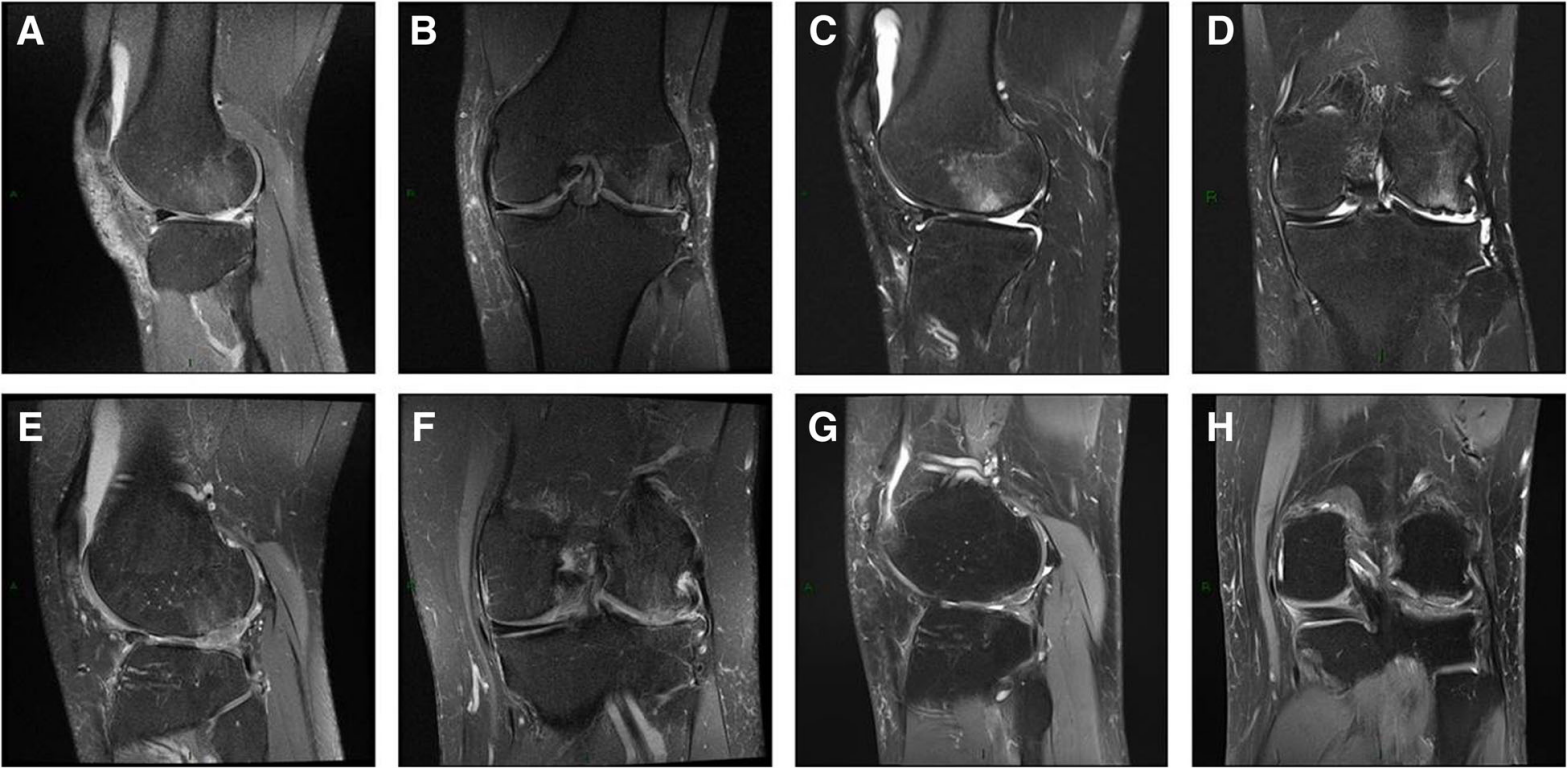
MRI post-surgery. Patient SPC: a sagittal fat-supressed proton density-weighted image 3 months after treatment. The graft tissue homogeneous filling the defect. Subchondral marrow edema. b Coronal fat-suppressed proton density-weighted image 3 months after treatment. Graft tissue homogeneous filling the defect. There is subchondral bone marrow edema, C: Sagittal fat-suppressed proton density-weighted image 53 months after treatment. The repair site is now filled with a congruent articular surface. Normal signal of the cartilage. Persistent bone marrow. d Coronal fat-suppressed proton density-weighted image 53 months after treatment. The repair site is now filled with a congruent articular surface. Persistent bone marrow edema and a focal irregularity in superficial layer are present. Patient CRC: e Sagittal fat-suppressed proton density-weighted image 6 months after the index procedure. The graft tissue inhomogeneous fills the defect with overgrowth of repair cartilage. Subtle subchondral marrow edema. f Coronal fat-suppressed proton density-weighted image 6 months after treatment. There is graft tissue in homogeneously filling the defect with overgrowth of the repaired cartilage. Subtle subchondral marrow edema. g Sagittal fat-suppressed proton density-weighted image 15 months after treatment. The repair site shows evidence of progression of tissue incorporation and a congruent articular surface. There is no bone marrow edema. h Coronal fat-suppressed proton density-weighted image 15 months after treatment. The repair site is now filled with incorporated tissue, and a congruent articular surface is evident. There is no bone marrow edema
